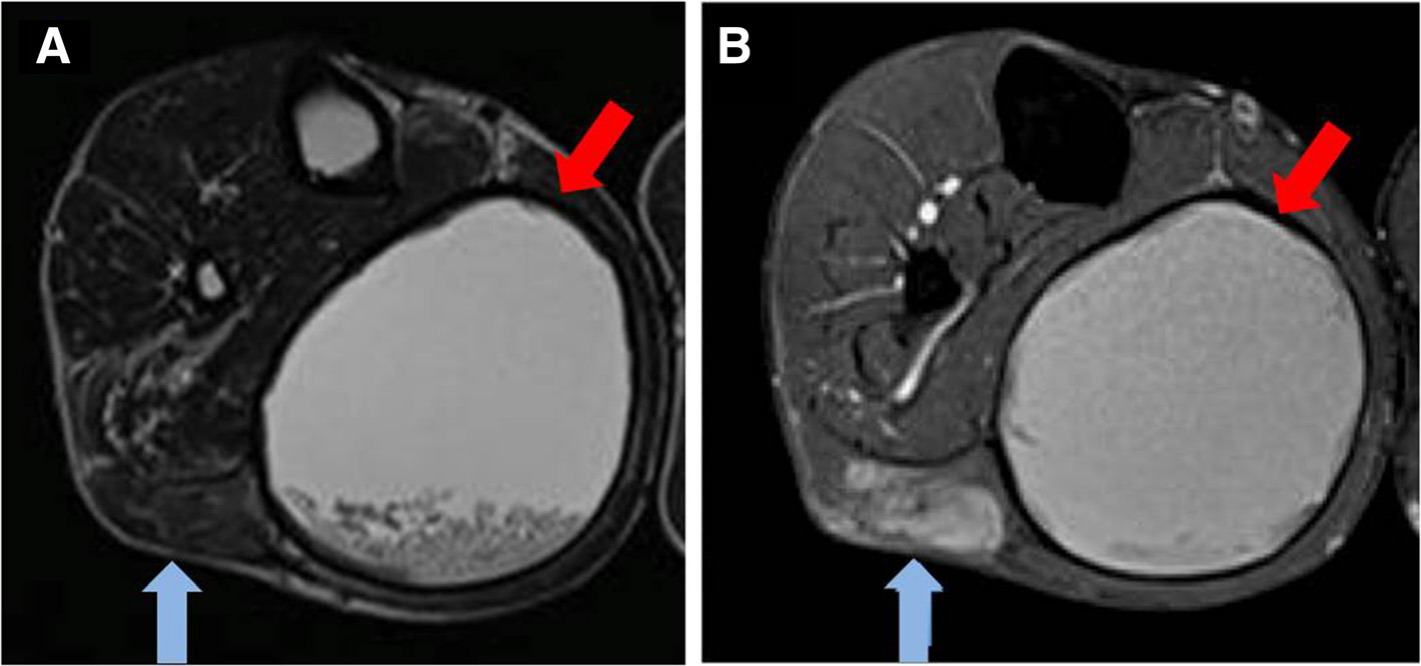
Magnetic resonance imaging scan. Axial magnetic resonance imaging (T2, Gd-enhanced) views show a well-defined cystic mass (red arrow) with debris in the right medial calf (A), and a Gd-enhanced solid lesion in the medial gastrocnemius (blue arrow) (B).
